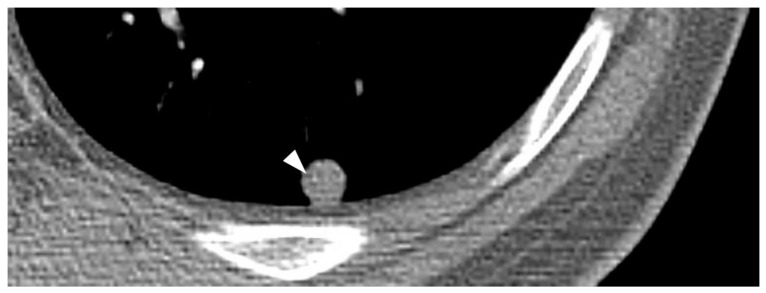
Pulmonary metastasis from synovial sarcoma containing tiny calcification. Precontrast CT image shows small metastatic synovial sarcoma in the lung and this nodule contains tiny calcification (arrowhead).
